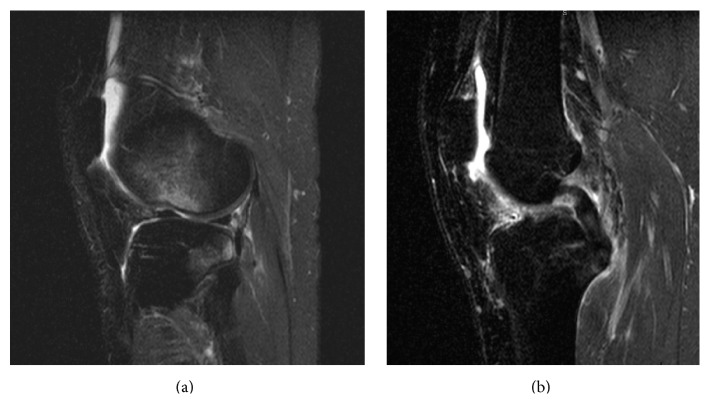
T2 weighted fat suppressed sagittal plane MRI images for evaluation of acute knee injury. Classic marrow contusion pattern at the lateral terminal sulcus and posterior aspect of the lateral tibial plateau (a) and complete rupture of anterior cruciate ligament (b).
